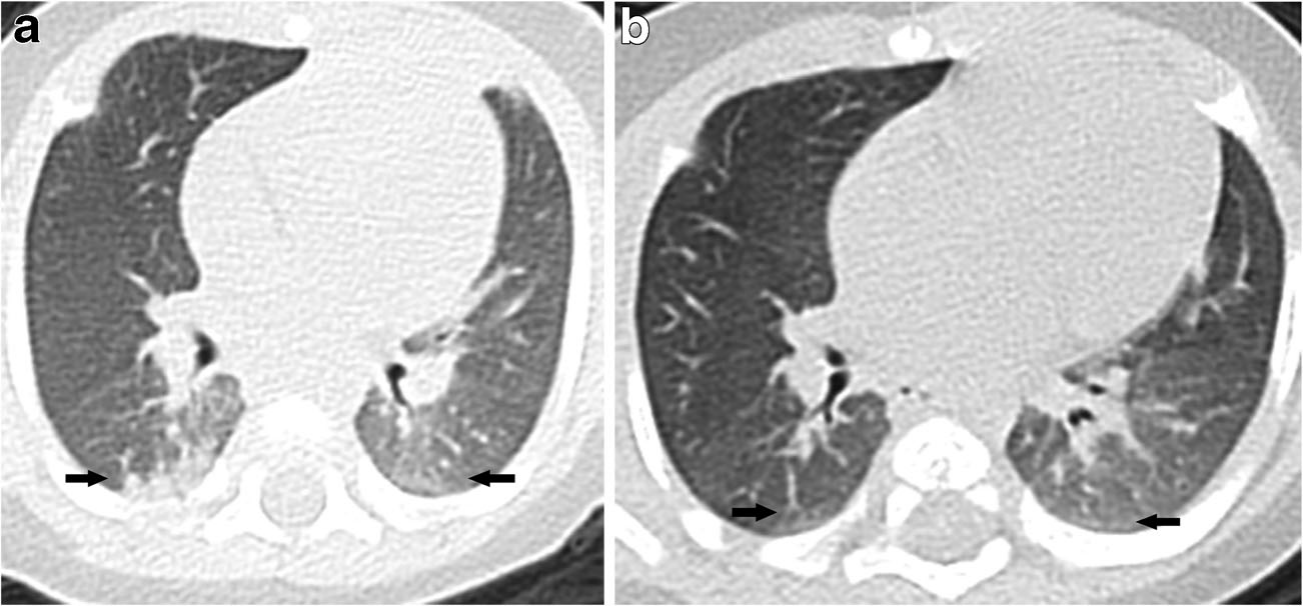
A 2-month-old symptomatic girl with COVID-19 and a history of definite contact presented with paroxysmal cough and rhinorrhea. a Axial thin-section non-contrast CT shows ground-glass opacities and consolidation in the subpleural zone of both lower lobes (*arrows*). b A follow-up CT image shows the lesions are markedly absorbed after 1 month (*arrows*)
